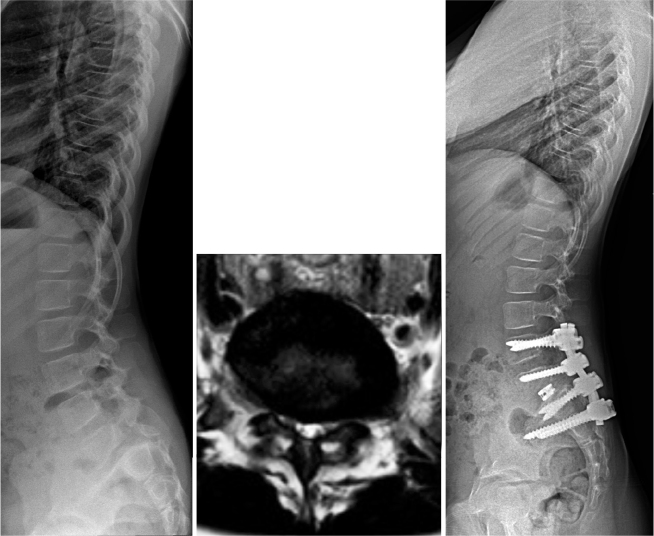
High-grade spondylolisthesis in a 9-year-old girl. (A) Standing lateral radiograph shows pelvic retroversion (increased pelvic tilt) and lower thoracic lordosis as a sign of compensatory mechanisms for sagittal imbalance. (B) Axial T2 weighted MR image shows central canal stenosis typical of dysplastic high-grade spondylolisthesis. (C) Instrumented spinal fusion from L4 to S1 was supplemented by iliac screws to prevent bending of sacrum between S1 and S2 disc space. Reduction was facilitated by sacral dome osteotomy and interbody fusion was carried out to increase the possibilities for circumferential spinal fusion.
